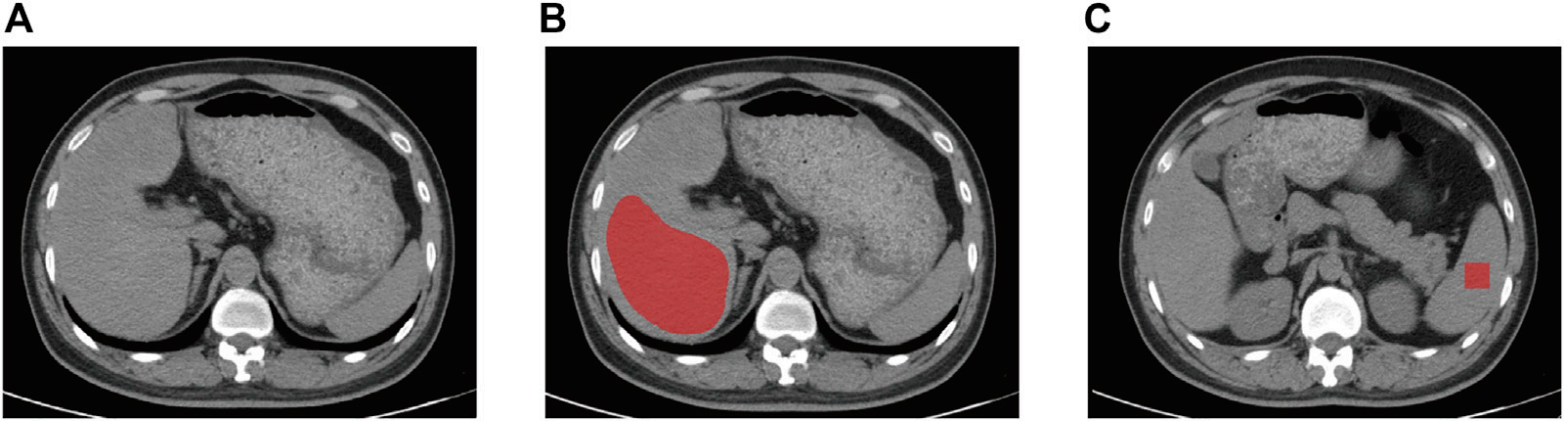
Non-contrast CT image of a 42-year-old man with mild liver steatosis. The ROI of liver (A) and spleen (B) is indicated by the red area. The ROI of liver was delineated along the margin of the right hepatic lobe at the level of right portal vein by avoiding large hepatic vessels and the ROI for spleen is 4 cm^2^ square of two contiguous sections. CT, computed tomography, ROI, region of interest.
